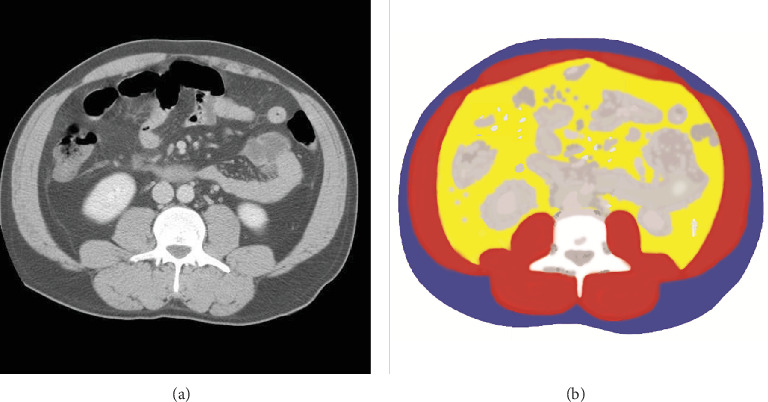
Abdominal CT image (a) and corresponding segmented image (b) separated into three main regions (subcutaneous fat colored blue, muscle colored red, and visceral fat colored yellow) with bone (white) and other tissues (gray).
